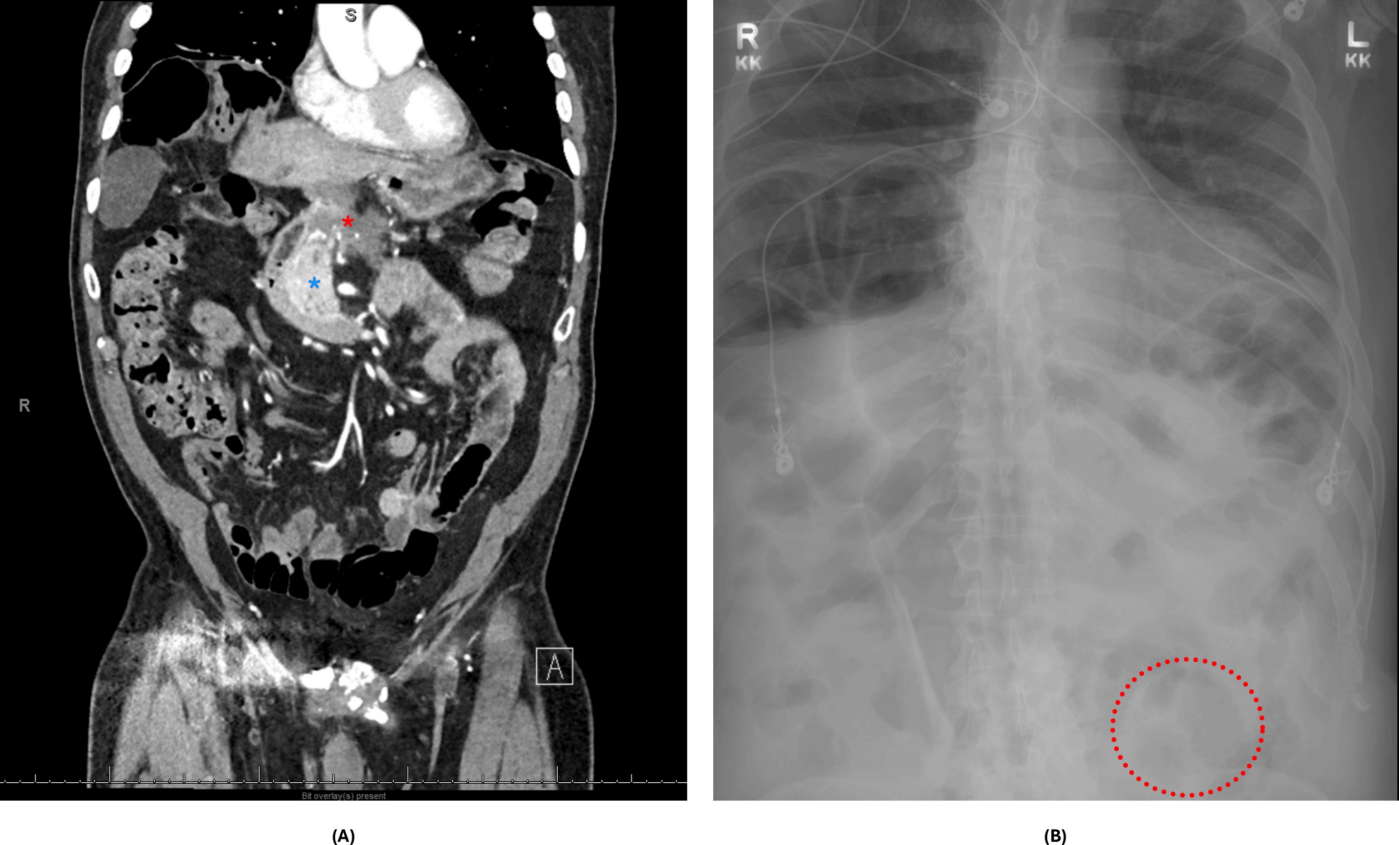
(A) CT imaging revealed increased soft tissue around the distal pancreatectomy site, concerning for malignancy. (B) An abdominal radiograph obtained post-endoscopic detorsion showed ongoing, re-demonstrated volvulus. (A) The blue star denotes the pancreas. The red star denotes a relatively hypo-attentuated area suggestive of soft tissue mass. The small hyper-attenuated spots in the mass are surgical clips from the previous distal pancreatectomy. (B) The red circle highlights the "coffee-bean sign," which is a radiologic sign of sigmoid volvulus.
